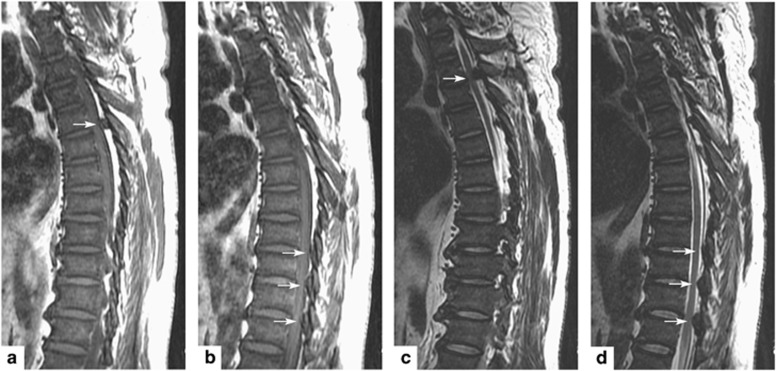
Sagittal image of hypointensity magnetic resonance imaging shows hypertrophy ligamentum flavum. (a) T1-weighted image shows the lesion at the T3-T4 level. (b) T1-weighted image shows the lesions at the T9-T10, T10-T11 and T11-T12 levels. (c) T2-weighted image shows the lesion at the T3-T4 level. (d) T2-weighted image shows the lesions at the T9-T10, T10-T11 and T11-T12 levels.
